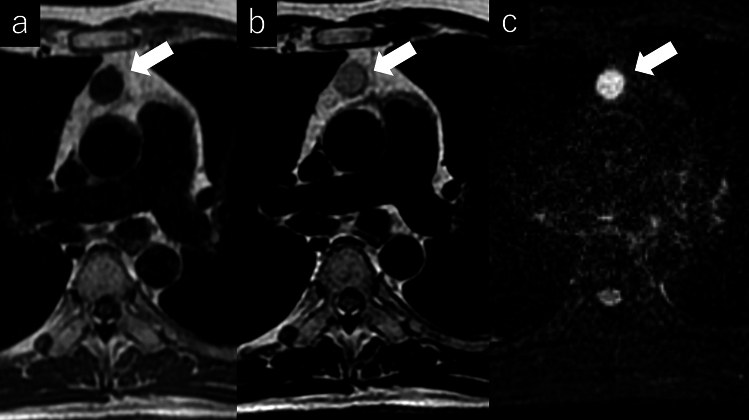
Thymic cyst. A 51-year-old man with thymic cyst. A 2-cm round cyst is observed in the anterior mediastinum. a T1WI shows low signal intensity (arrow). b T2WI shows a slightly high signal intensity (arrow). c Fat-suppressed T2WI shows a markedly high signal intensity (arrow). The cyst is a well-defined, unifocal lesion with serous content. T1WI, T1-weighted image; T2WI, T2-weighted image
